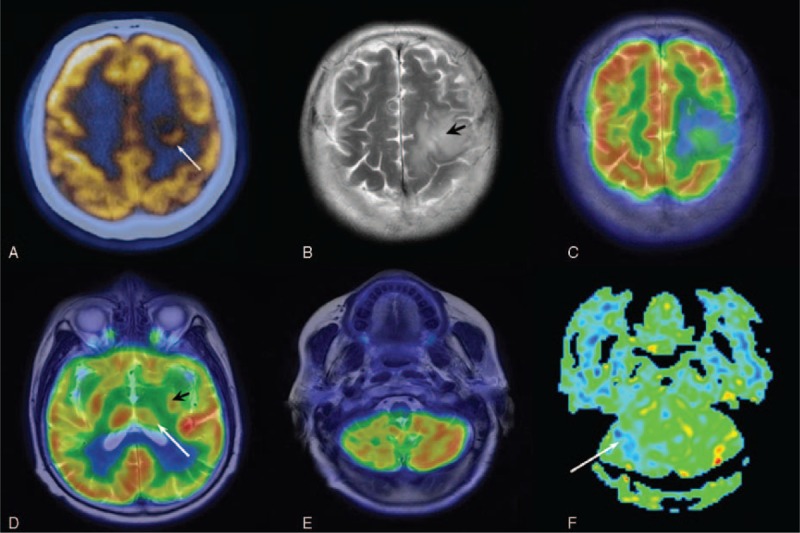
A 73-year-old woman confirmed the diagnosis of brain metastases. (A) Axial fused PET/CT showed a ring-like area of 18F-FDG uptake in the left frontal lobe with ill-defined margin (arrow). (B) Axial FSE T2WI exhibited in the left frontal lobe an area of high signal suggestive for perilesional edema (arrowhead). (C) Axial FDG PET fused with FSE T2WI showed the corresponding peritumoral edema with hypometabolism. (D) The axial FDG PET/MRI image of this patient showed hypometabolism in the homolateral basal nuclei (arrowhead) and thalamus (arrow). (E) Fused PET/MRI images show a marked reduction in cortical and subcortical glucose metabolism in the right cerebellar hemisphere. (F) The ASL perfusion image done at the same time also showed the right cerebellar hypoperfusion (arrow), in keeping with the phenomenon of CCD. ASL = arterial spin-labeling, CCD = crossed cerebellar diaschisis, FDG = fluorodeoxyglucose, MRI = magnetic resonance imaging, PET = positron emission tomography.
